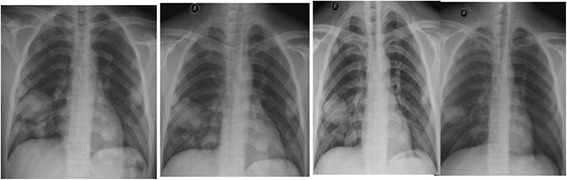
Multiple chest x-rays showing disease progression over time
